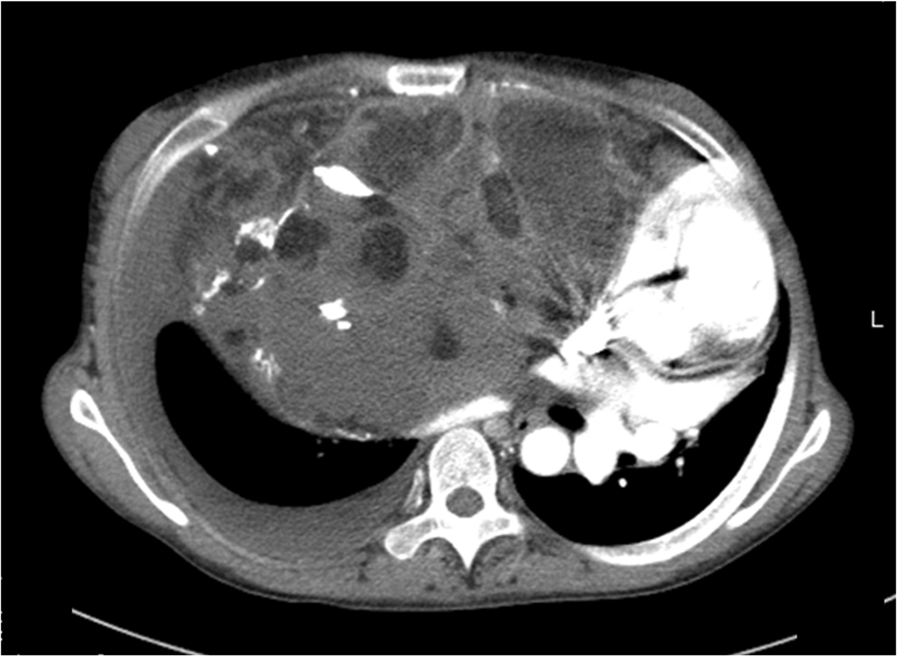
Preoperative computed tomography scan of the abdomen showing a giant solid mass.
